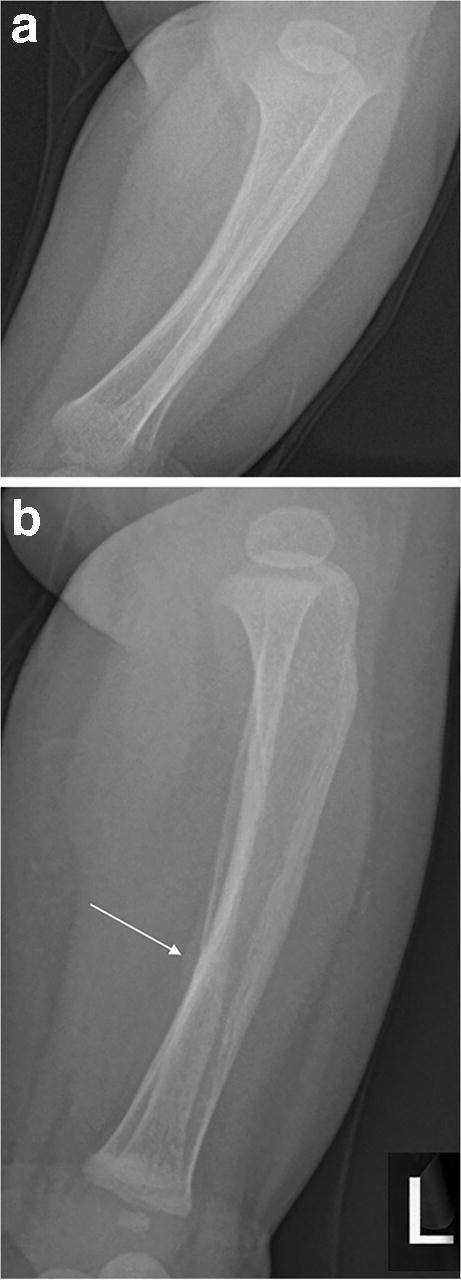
A 6-month-old boy with healing fractures of both fibulae. a–b Anteroposterior (a) and lateral (b) radiographs (cropped and magnified) of the left tibia and fibula. There is subtle periosteal reaction along the posterior cortex of the fibular shaft at the junction of middle and distal thirds (*arrow*). A faint horizontal line runs through the posterior cortex of the fibula, centred on the periosteal reaction and suggests an undisplaced fracture. Images were initially reported as normal
